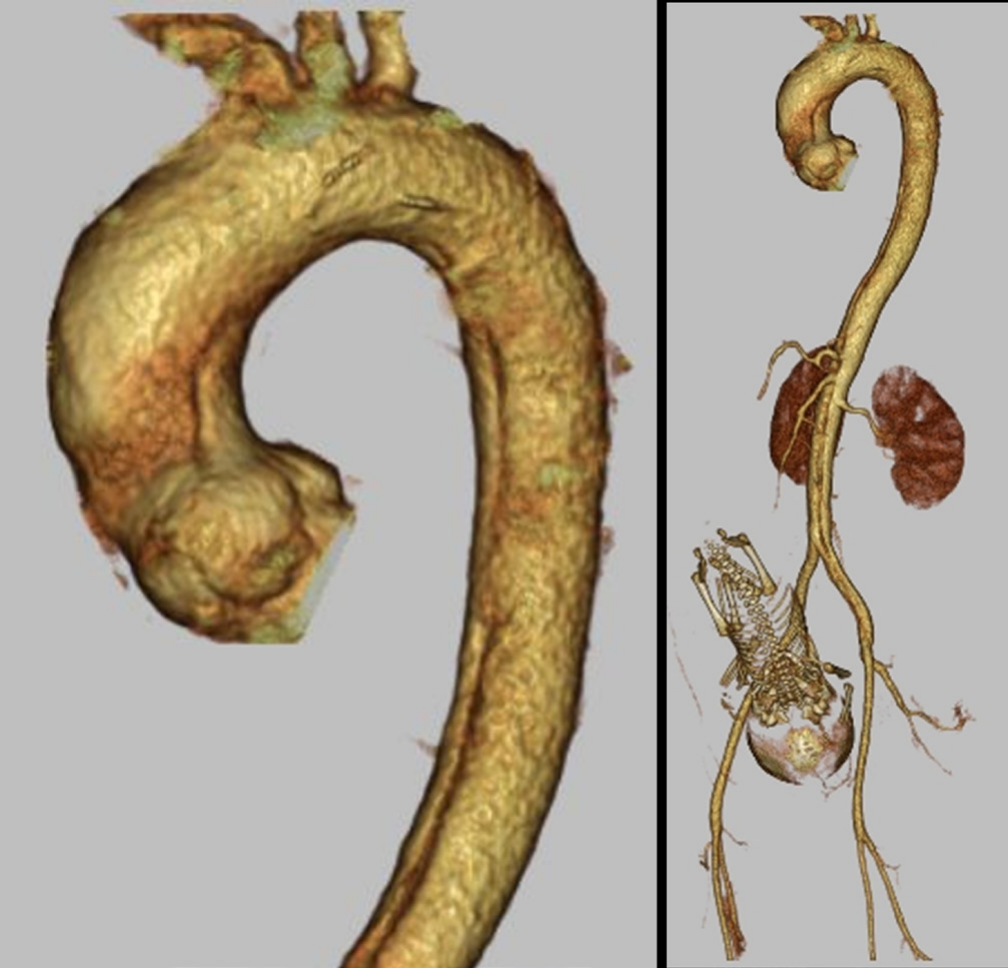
Preoperative computed tomography image Acute type A aortic dissection
